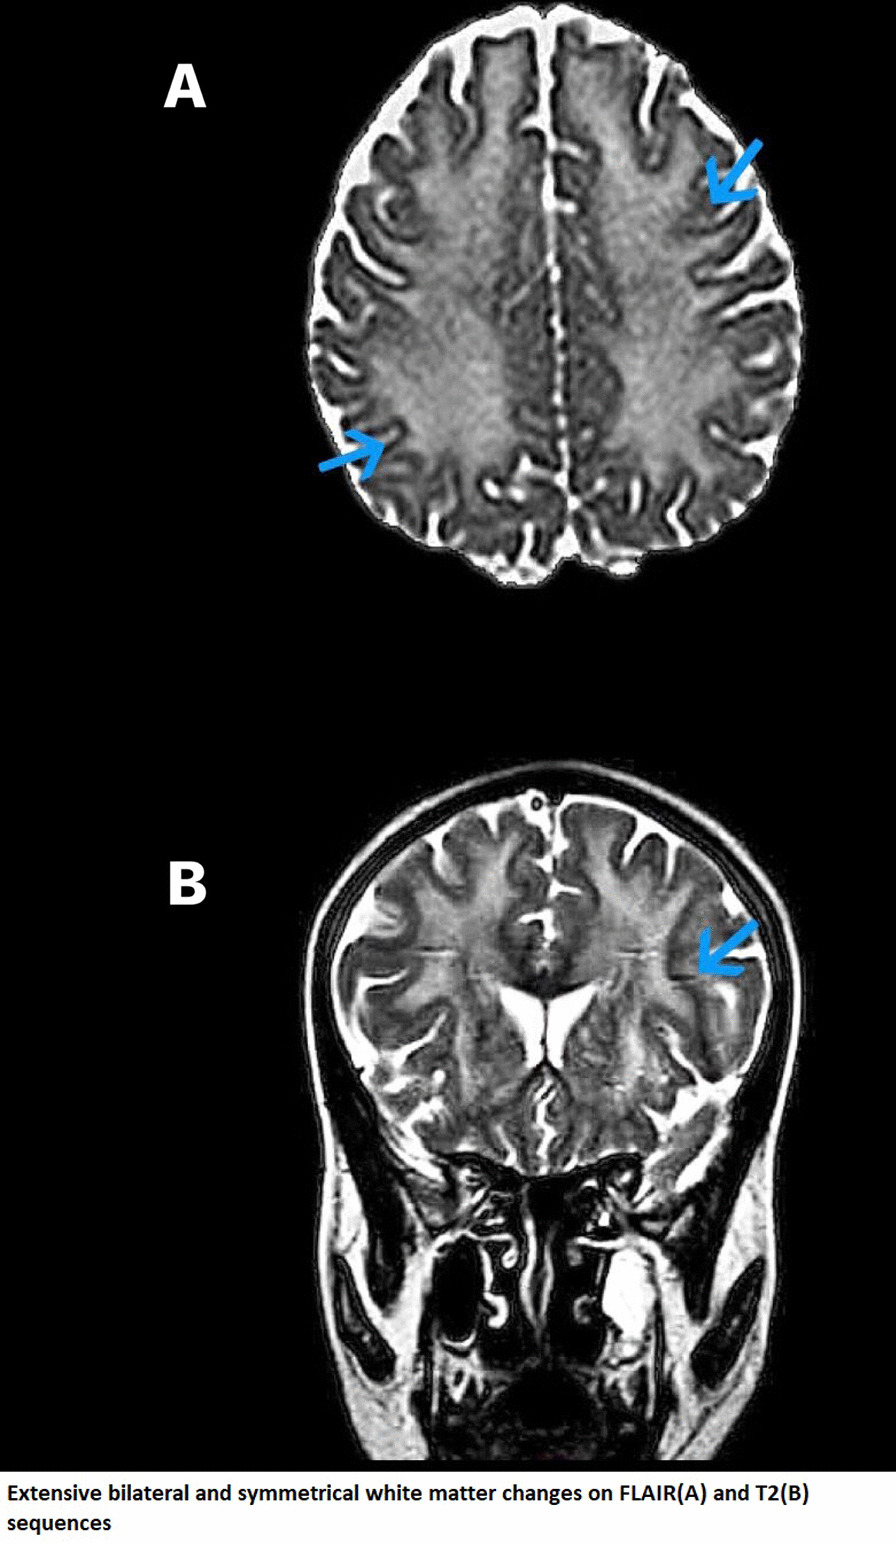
Magnetic resonance imaging brain showing T2 and flair sequences. The arrow points out to demyelinating white matter changes in T2 and flair sequences
